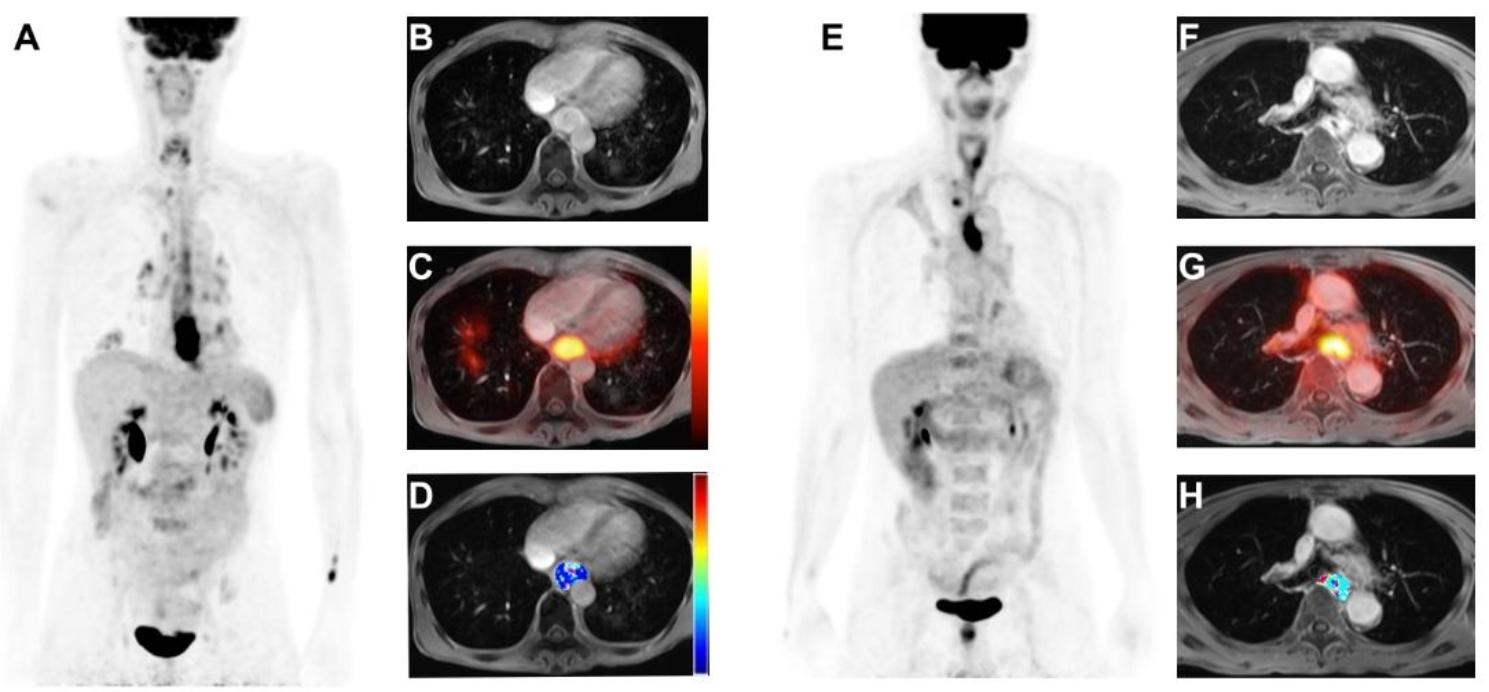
A combination of PET and MRI functional biomarkers in predicting overall survival. (A–D) Representative axial images of a lower-third esophageal cancer patient with T3N2 disease. (A) Interim maximal intensity projection PET image; (B) Interim contrast-enhanced MR image; (C) Fused interim [18F]FDG-PET/MRI image; and (D) Interim contrast-enhanced MR image with an overlaid *Ve* map of the primary tumor. This patient had an interim TLG of 113 and an interim *Ve* value of 34. He died of tumor recurrence with a short overall survival period of 8 months after definitive chemoradiotherapy. (E–H) Images of an upper-third esophageal carcinoma patient with T4bN2 disease. (E) Interim maximal intensity projection PET image; (F) Interim contrast-enhanced MR image; (G) Fused interim [18F]FDG-PET/MRI image; and (H) Interim contrast-enhanced MR image with an overlaid *Ve* map of the primary tumor. He had an interim TLG of 45 and a *Ve* value of 162. This patient still survived without disease recurrence for four years after definitive chemoradiotherapy
